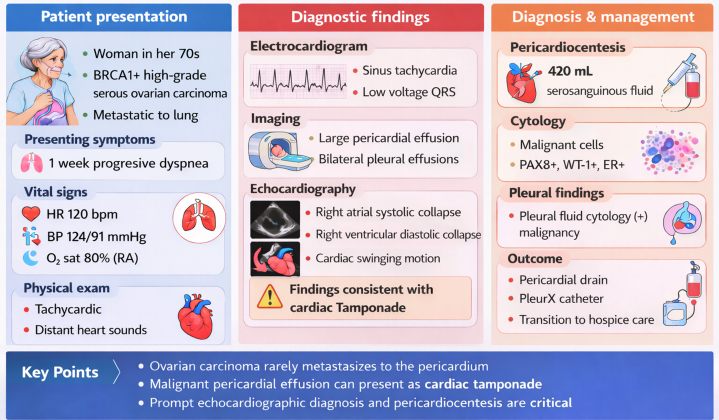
A Woman in Her 70s With BRCA1-Positive High-Grade Serous Ovarian Carcinoma Metastatic to the Lung Presented With Progressive Dyspnea, Tachycardia, Hypoxemia, and Distant Heart Sounds Echocardiography revealed cardiac tamponade. Pericardiocentesis yielded 420 mL of serosanguinous fluid; cytology demonstrated malignant cells positive for PAX8, WT-1, and ER, confirming pericardial metastasis. The case highlights rare pericardial metastasis and the need for rapid recognition and intervention. BP = blood pressure; BRCA1 = breast cancer gene 1; ER = estrogen receptor; HR = heart rate; PAX8 = paired box gene 8; RA = room air; WT-1 = Wilms tumor 1.
